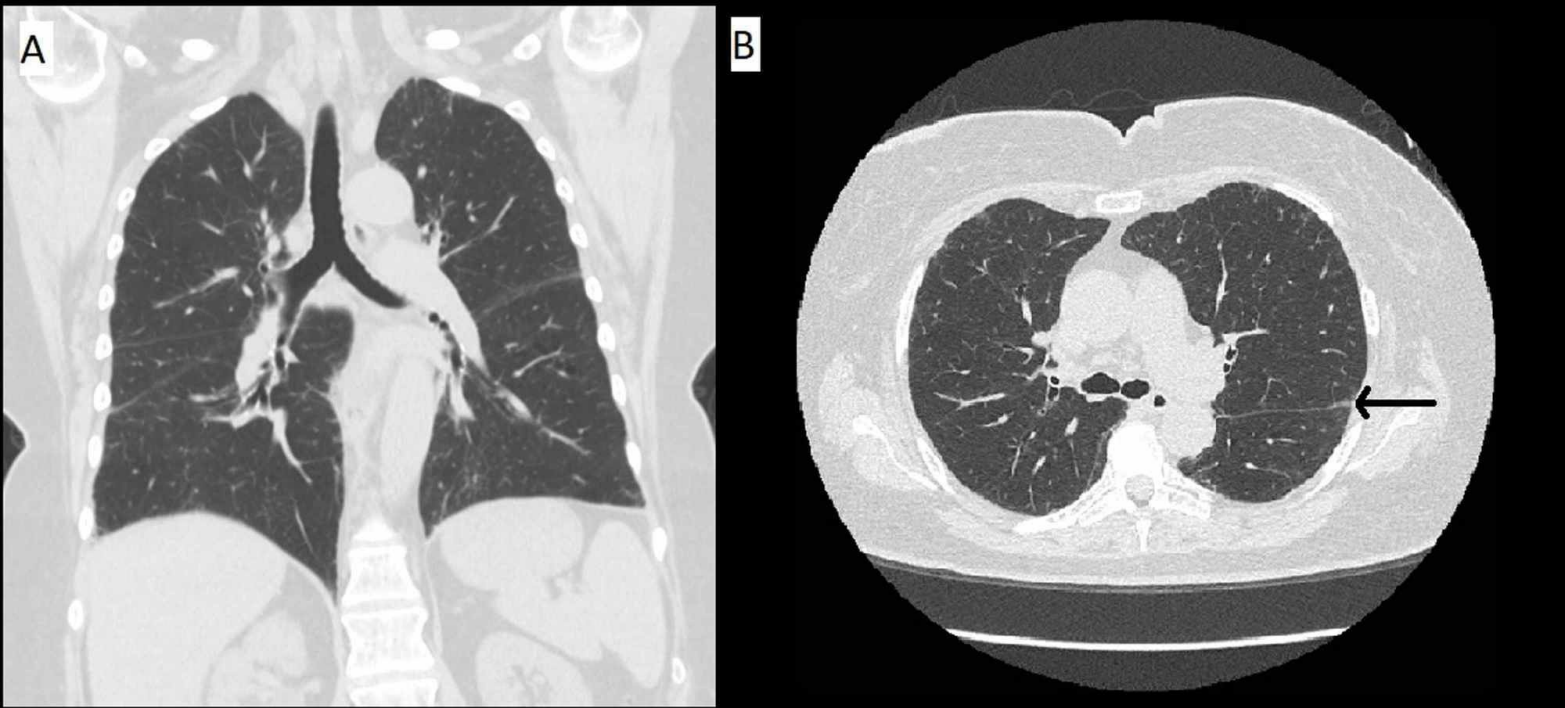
CT imaging nine months after presentation Coronal CT chest imaging (A) and axial CT chest imaging (B) showing continued improvement of parenchymal disease with only subtle residual reticulations and very minimal groundglass (black arrow).
